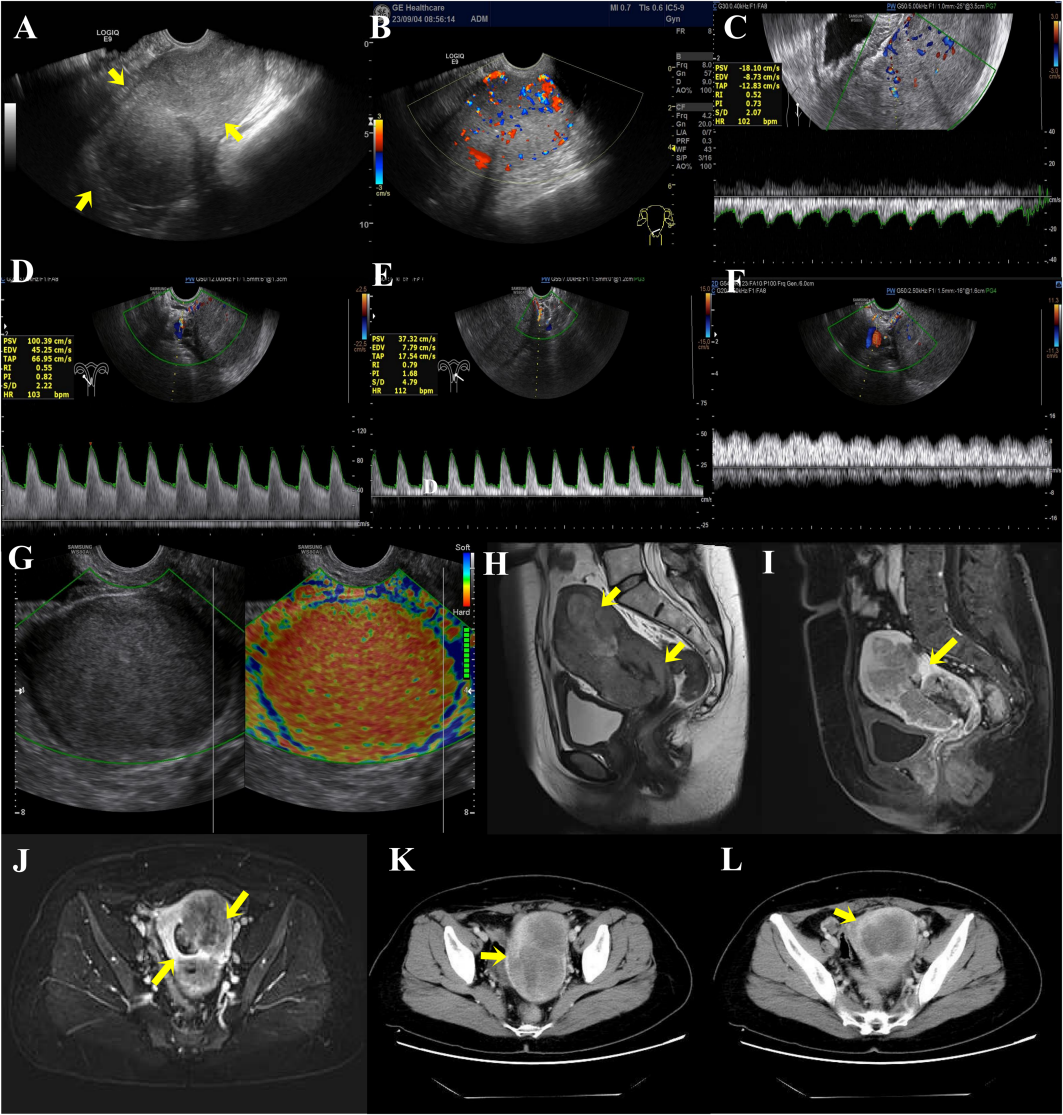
(A) Preoperative transvaginal color Doppler ultrasound sonogram of endometrial cancer lesions. Full uterine morphology, unclear cervical and uterine structures, and diffuse lesions in the myometrium (arrows show the location of infiltration of lesions). (B–G) Blood flow in and around the lesion. (B) Color Doppler ultrasound showed abundant blood flow signals in the interior and margins of the lesion. (C) Spectral Doppler ultrasound showed a low resistance type arterial flow spectrum detected within the lesion. RI: 0.52 (The general resistance index RI is less than 0.4, whereas the RI of this case is higher.) (D) The right uterine artery has increased blood flow and decreased vascular resistance. (E) Decreased vascular resistance of the left uterine artery. (F) Spectral Doppler ultrasound defined that the veins next to the uterus show a blood flow frequency spectrum like pulsation. (G) Transvaginal ultrasonography E-cervix elastosonography shows the lesion area in red, representing hard tissue. (H–J) MRI scanning and MRI enhancement. (H) Uneven abnormal signal mass in the cervix and left lateral wall of the uterus. (I) Uneven enhancement in the lesion of the myometrium and uterine cavity. (J) The local endometrial structures are poorly demonstrated, and the intrauterine lesion is poorly demarcated from the myometrial lesion. (K, L) CT plain scan and CT enhancement on the whole abdomen. (K) The uterus is enlarged in shape, with marked heterogeneous enhancement of the myometrium, predominantly in the left wall. (L) The lesion is poorly demarcated from the uterine cavity and endometrium.
